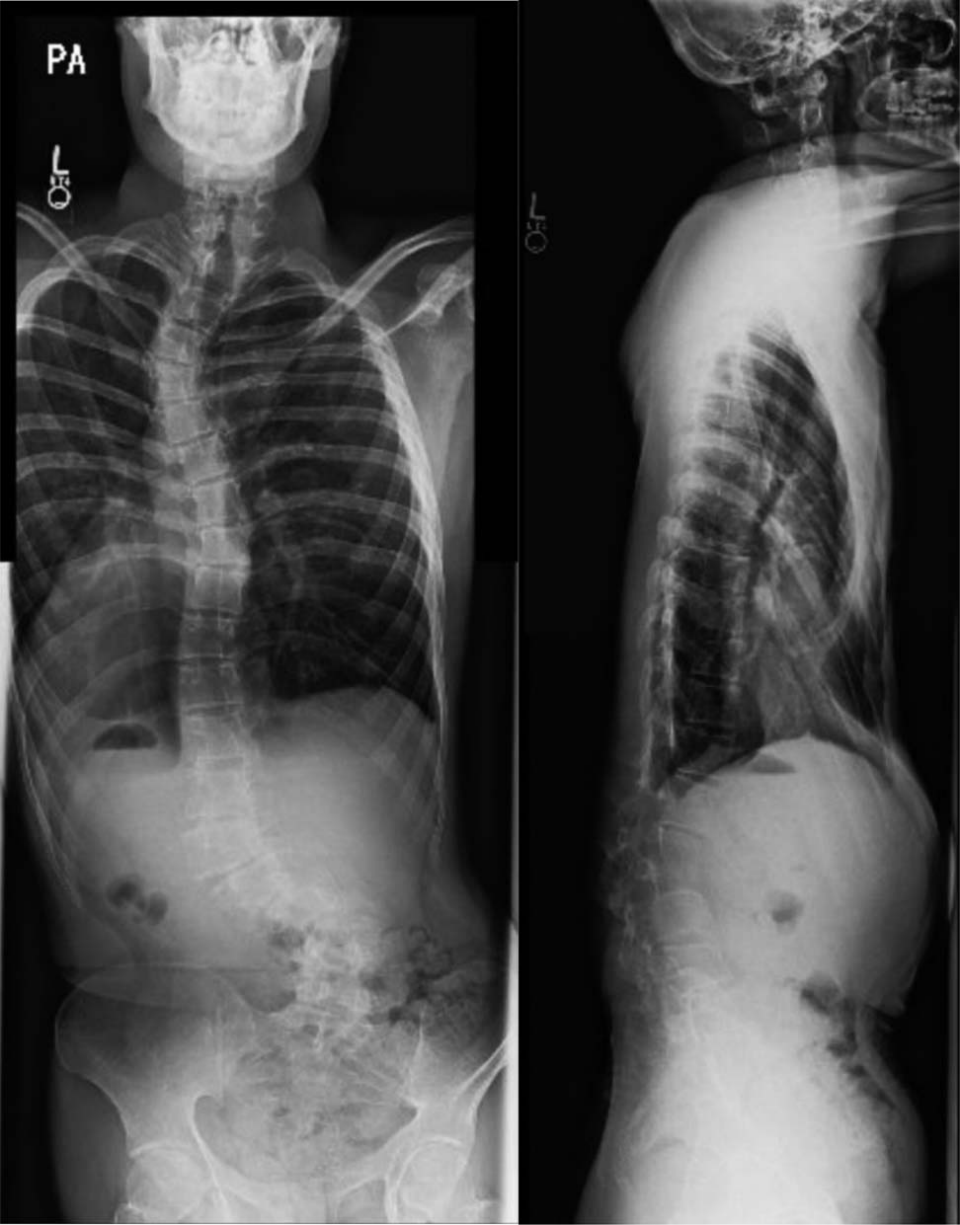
Posteroanterior and lateral plain radiographs of the initial presentation demonstrating lumbar kyphosis and leftward listhesis of L2-3 and L3-4.
